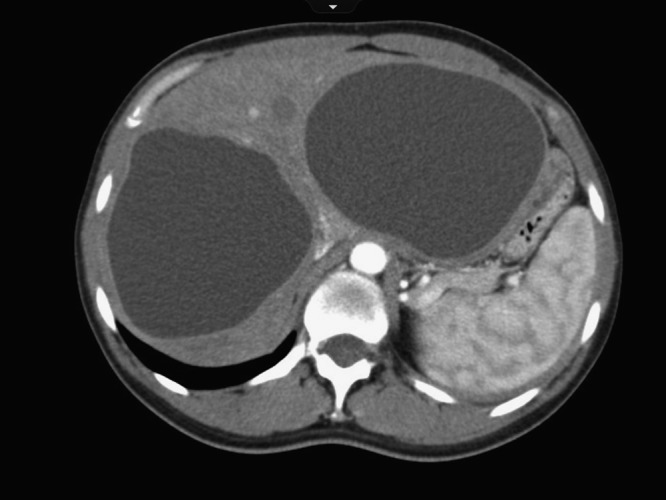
Computed tomography (CT) image of two large, unilocular hepatic hydatid cysts.
